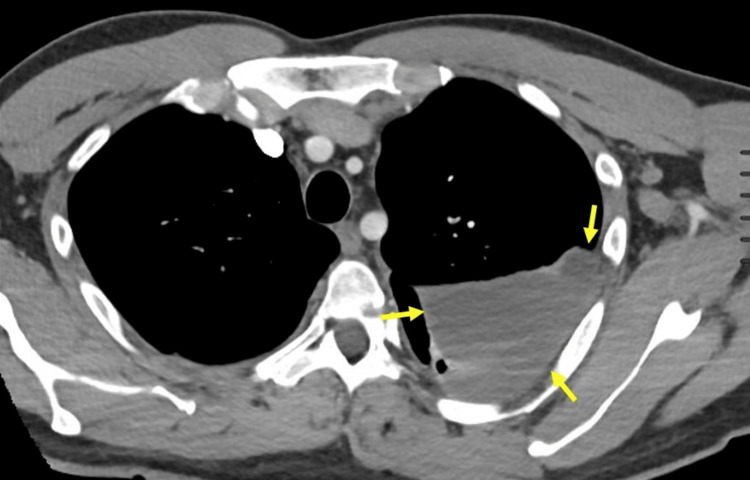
A non-contrast thoracic CT scan demonstrates a large left-sided loculated pleural effusion (yellow arrows) CT: computed tomography
